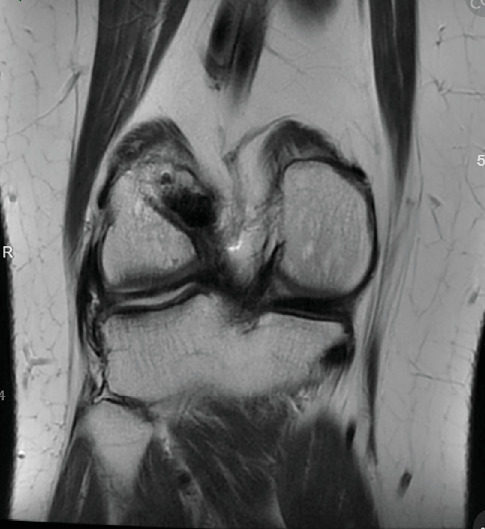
MRI from 09/2022 demonstrating fill of the lateral tibial plateau cartilage defect.
